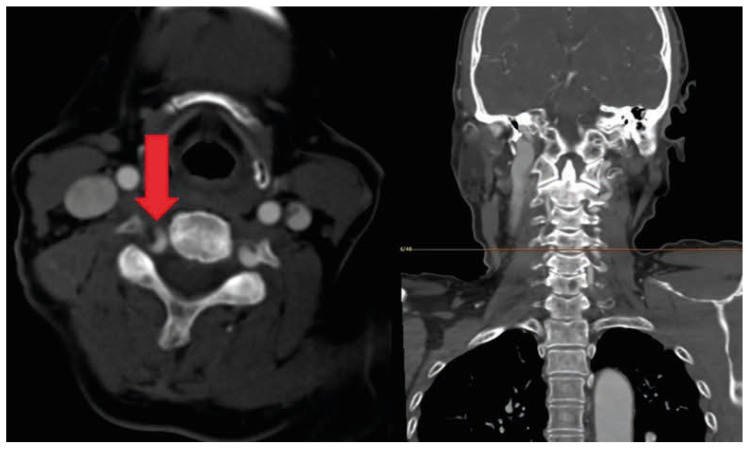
Neck computed tomography angiography demonstrating the vertebral artery coursing into and compressing the right C4-5 neural foramen (Red arrow).
